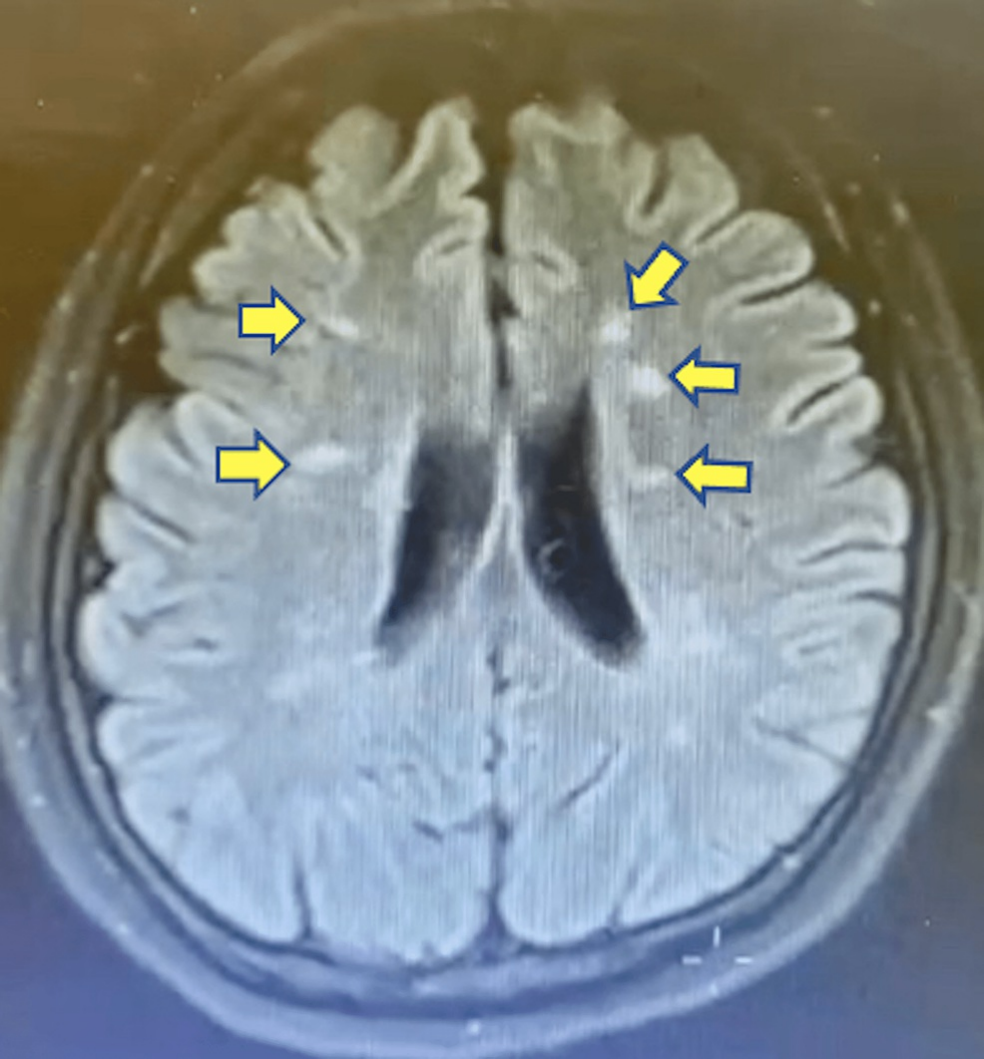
Axial view of an MRI FLAIR of the brain showing multiple periventricular white matter lesions (arrow) with Dawson's fingers appearance FLAIR: Fluid-attenuated inversion recovery
